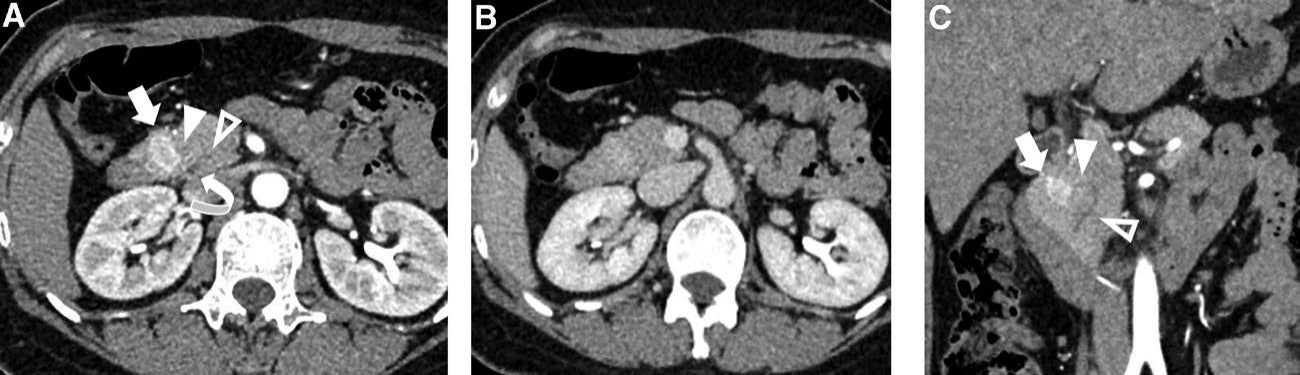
Contrast-enhanced CT “pNETs protocol.” (a) Axial early arterial phase image of 1-mm thickness revealed a hyper-enhancing nodule within the pancreatic head, indicating the tumor (white arrow). The white arrowhead, hollow arrowhead and curved arrow indicates the duct of Santorini, the duct of Wirsung and the common bile duct, respectively. (b) Axial portal venous phase image, the tumor nodule showed quick wash-out of contrast agent and became only slightly hyper-enhancing compared to the adjacent pancreatic parenchyma. (c) Reconstructed coronal early arterial phase image. Besides the hyper-enhancing tumor nodule (white arrow), the duct of Santorini (white arrowhead) and the duct of Wirsung (hollow arrowhead) are more clearly visible, with the duct of Santorini in close proximity to the tumor. CT = computed tomography, pNETs = pancreatic neuroendocrine tumors.
